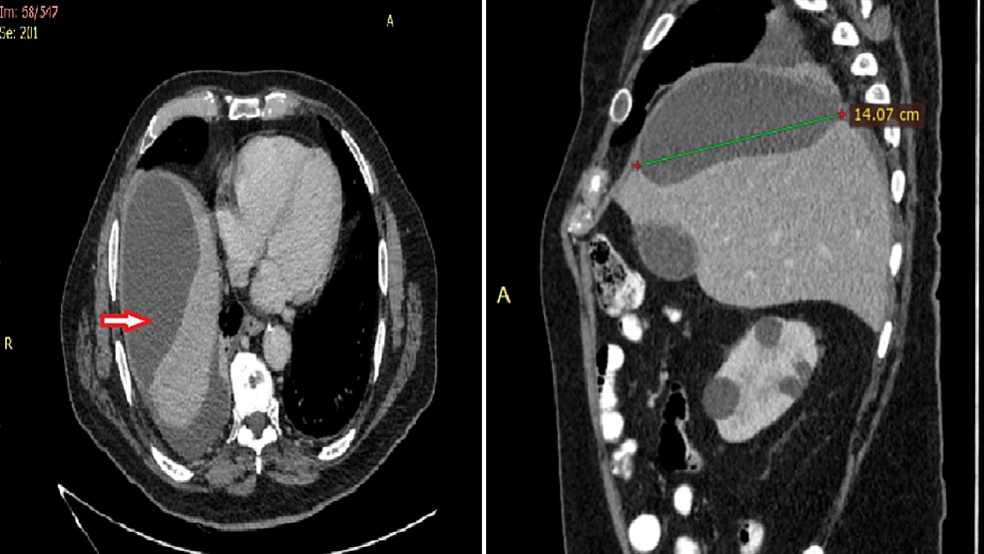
Abdominal CT images The arrow shows the subcapsular liver lesion in the sagittal plane. Its diameter is measured at about 14 cm.
